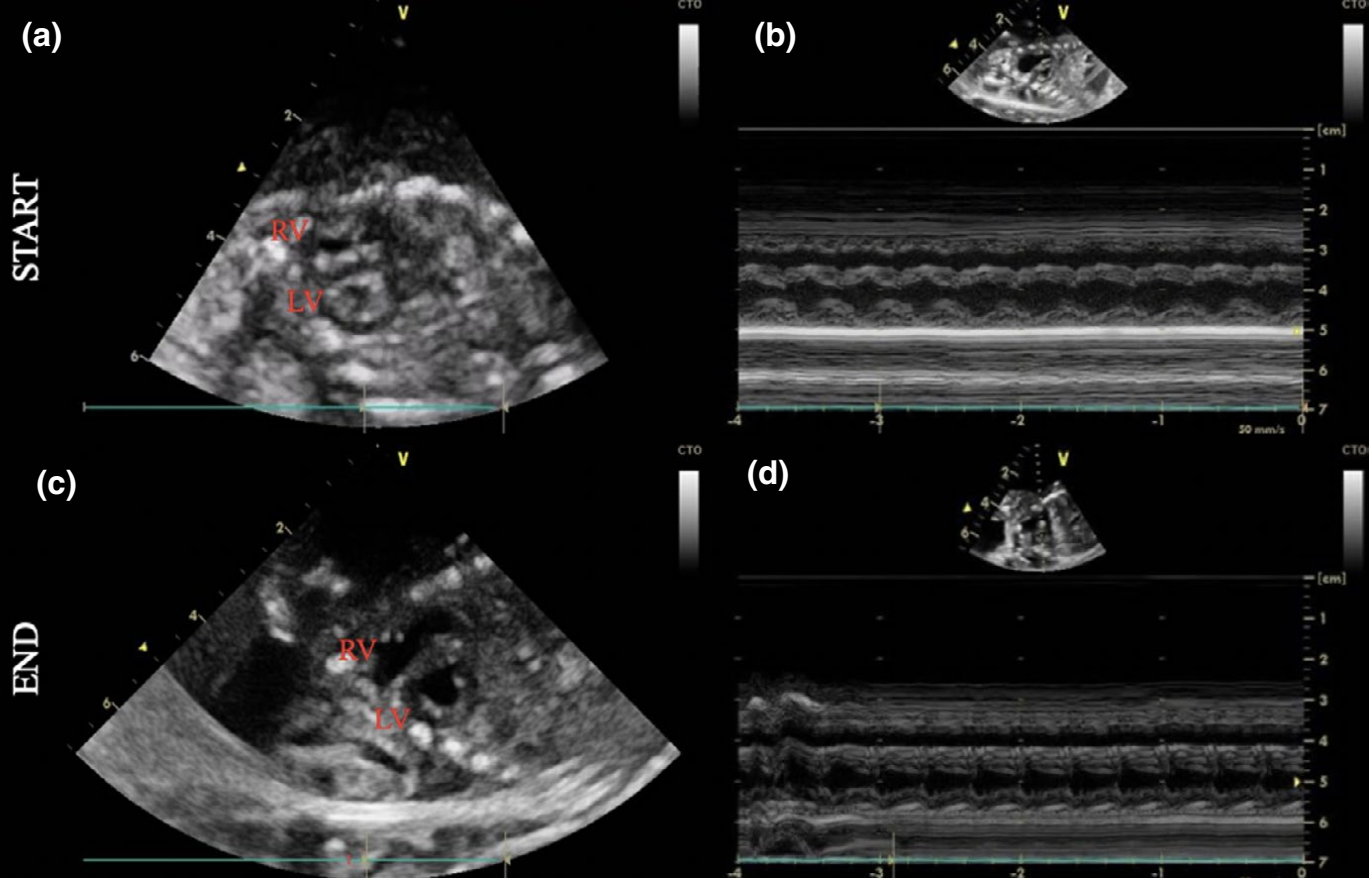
Fetal echocardiography at the start and end of the time on the AP circuit. Fetal echocardiography showing short‐axis view (a,c) and M‐mode (b,d) at the start (a,b) and end (c,d) of AP support. At the start of AP support, RV morphology and function were normal (a,b); however, at the end of AP support shows RV enlargement and diminished contractility (c,d)
